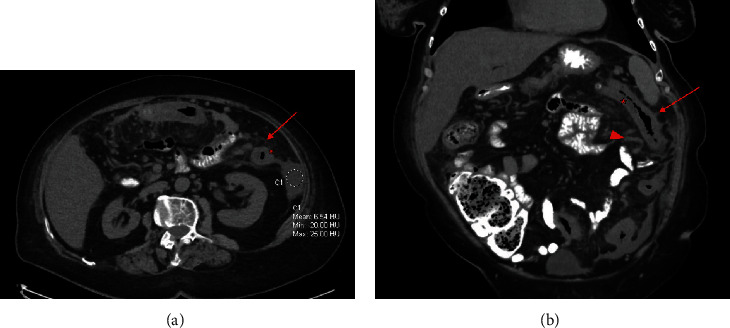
Initial presentation to the emergency department with diffuse abdominal pain and diarrhea. CT abdomen and pelvis with oral contrast only. Axial image (a) displays colonic mural thickening (star) and adjacent pericolonic fat stranding (arrow) most pronounced in the descending colon with ascites in the left paracolic gutter (circled). Coronal image (b) displays left hemicolon mural thickening (star) and adjacent pericolonic fat stranding (arrow) in the region of the IMA distribution. Suggestion of a prominent vascular structure is noted medial to the descending colon (arrowhead).
